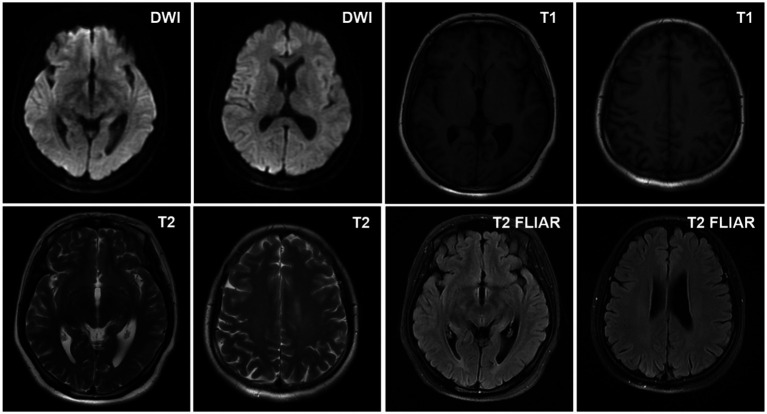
The representative images of brain magnetic resonance imaging (MRI) of the patient.
